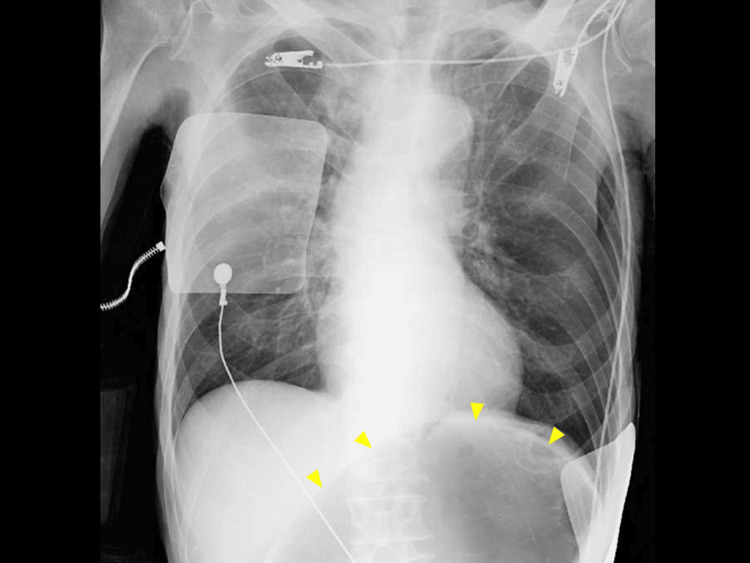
Chest radiograph It showed no chest lesions, but a colon distension beneath the diaphragm.
